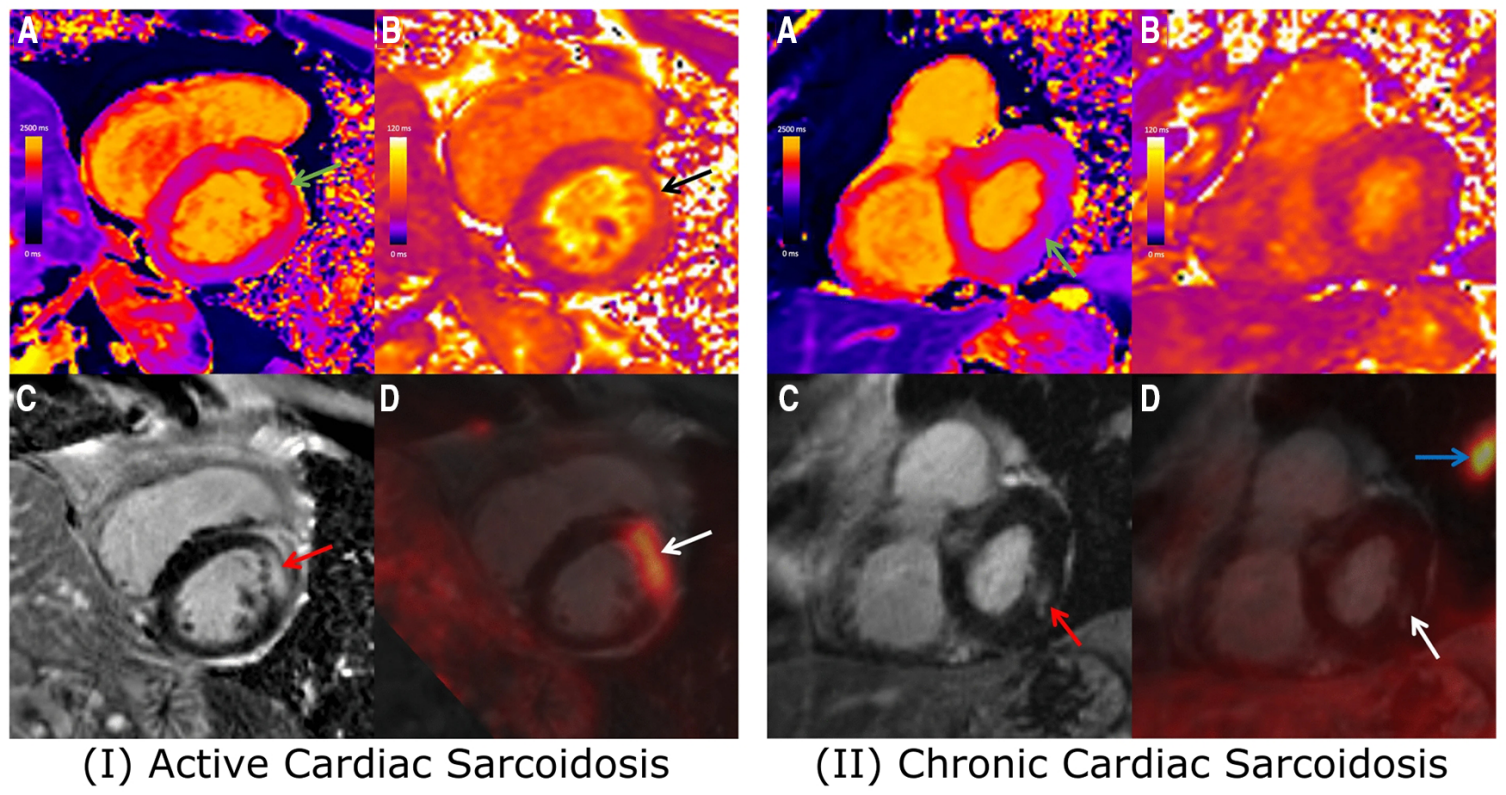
Combined ^18^F-FDG PET/MRI images showing short-axis slices including (A) native T1 map, (B) native T2 map, (C) LGE image, and (D) fused ^18^F-FDG PET and LGE image. (I) 67-year-old Male with cardiac and extra-cardiac sarcoidosis, with co-localized elevated T1 (green arrow), elevated T2 (black arrow), mid-wall LGE (red arrow) and focal FDG uptake (white arrow). With positive findings on both PET and MRI, the patient likely presents with active cardiac sarcoidosis. (II) 72-year-old Male with cardiac and extra-cardiac sarcoidosis, demonstrating slightly elevated T1 (green arrow) and corresponding mid-wall LGE (red arrow) at the inferolateral wall. No corresponding elevation of T2 or focal FDG uptake (white arrow) was observed, likely reflecting chronic, burnt-out cardiac sarcoidosis. *Adapted from Cheung, E., et al. (2021). Combined simultaneous FDG-PET/MRI with T1 and T2 mapping as an imaging biomarker for the diagnosis and prognosis of suspected cardiac sarcoidosis. European Journal of Hybrid Imaging*, *5*(1) and the original ahs a CC BY 4.0 license, available here: https://ejhi.springeropen.com/articles/10.1186/s41824-021-00119-w#rightslink.
